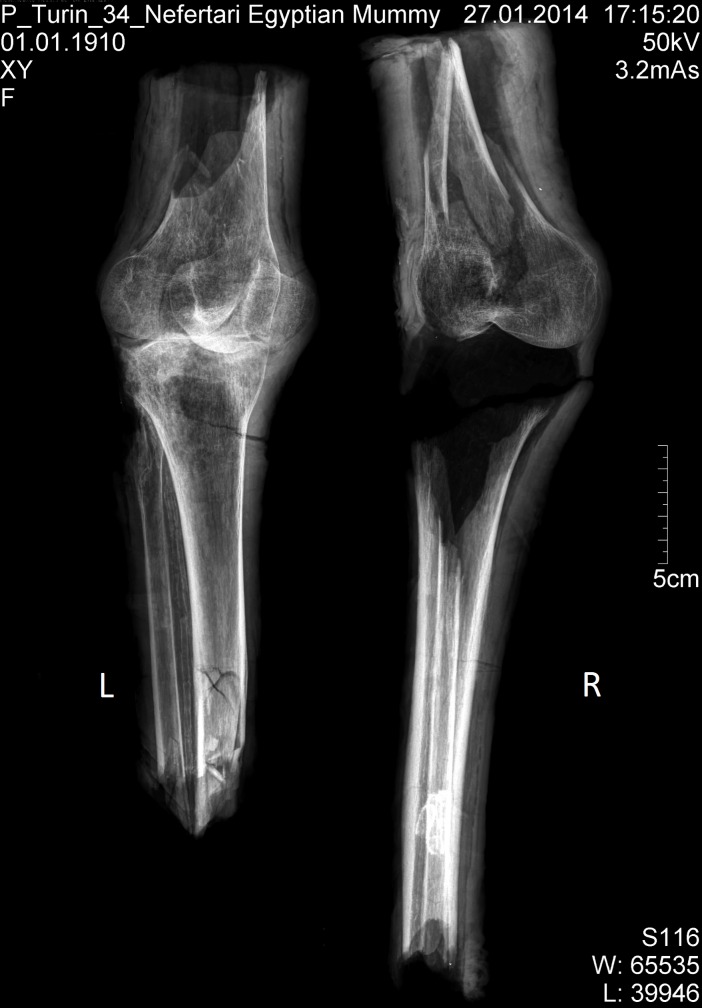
X-ray; arranged as seen in Fig 3.
